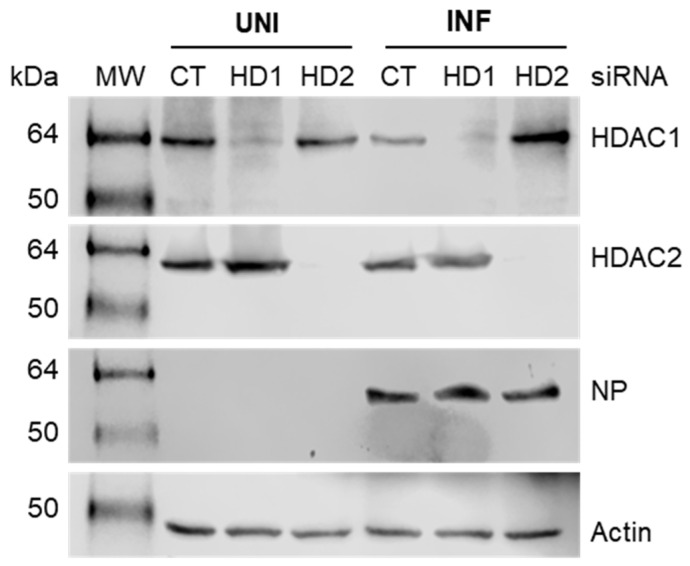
Western blots showing the siRNA-mediated depletion of HDAC1 and HDAC2 expression in uninfected and infected cells. HeLa cells were transfected with either control (CT), HDAC1 (HD1), or HDAC2 (HD2) siRNA for 30 h. Cells were then infected with PR8 at MOI of 3.0. After 6 h, HDAC1 (62 kDa), HDAC2 (60 kDa), NP (56 kDa), and Actin (42 kDa) were detected in total cell lysates by Western blotting. UNI, uninfected; INF, infected; kDa, kilodalton.
